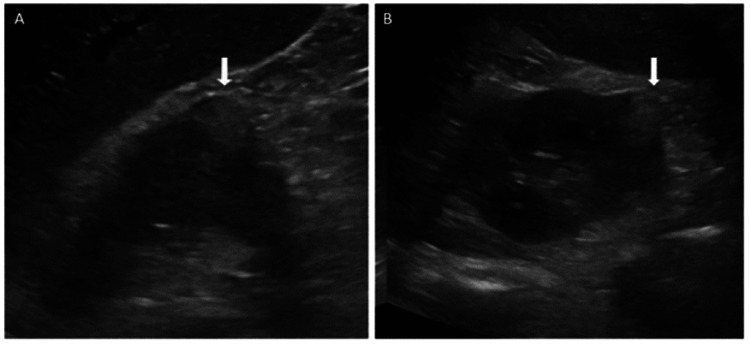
Follow-up imaging Renal ultrasound (A & B) of the right kidney showing a 2.5 x 2 x 2.2 cm oval density representing a resolving hematoma
